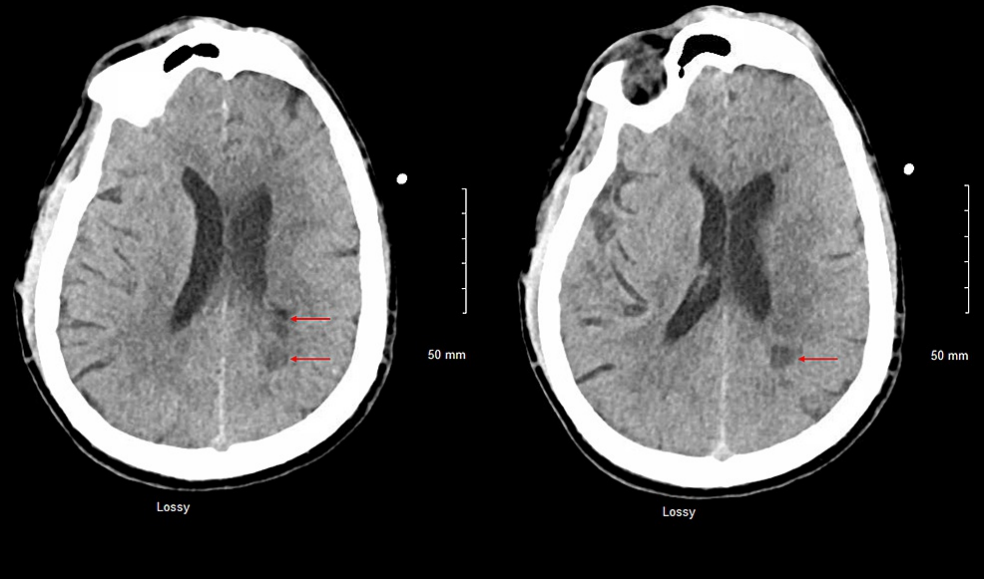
CT head axial view plain revealed multiple white matter hypodensities concerning septic emboli (Red arrows). CT: computed tomography
